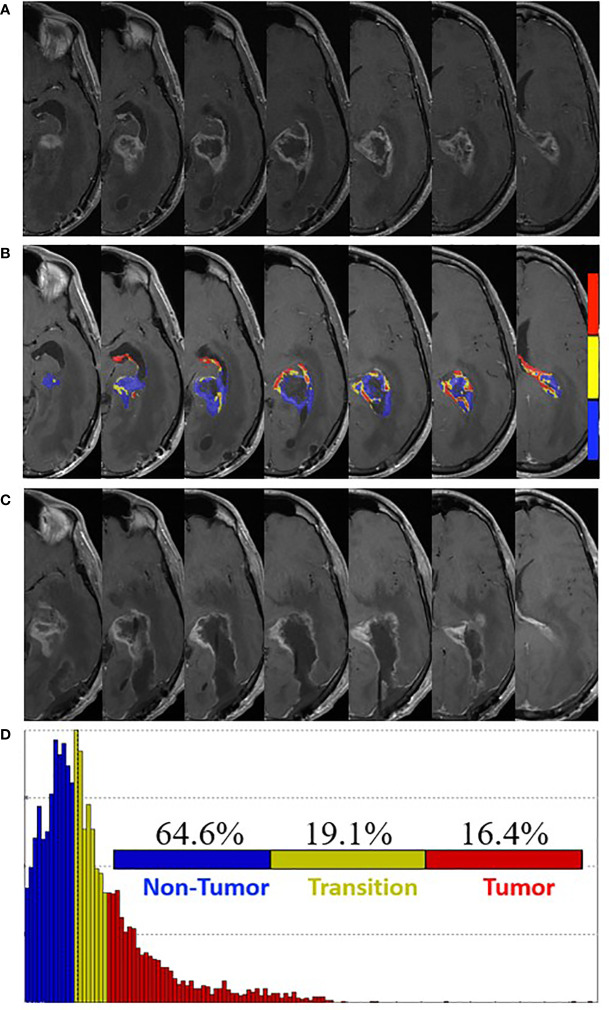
Pre- and post-surgery MRI. (A) Postcontrast T1-weighted MRI obtained four weeks post-pLDR treatment and one week before redo-surgery. (B) Corresponding maps of FTB superimposed on the postcontrast T1-weighted MRI images. The FTB maps show areas of active tumor (red), treatment effect (blue), and transitional zone (yellow). (C) Post-surgery (one week) postcontrast T1-weighted MRI demonstrating extent of resection. (D) Distribution of T1-weighted enhancement volume based on vascularization as measured by FTB maps.
